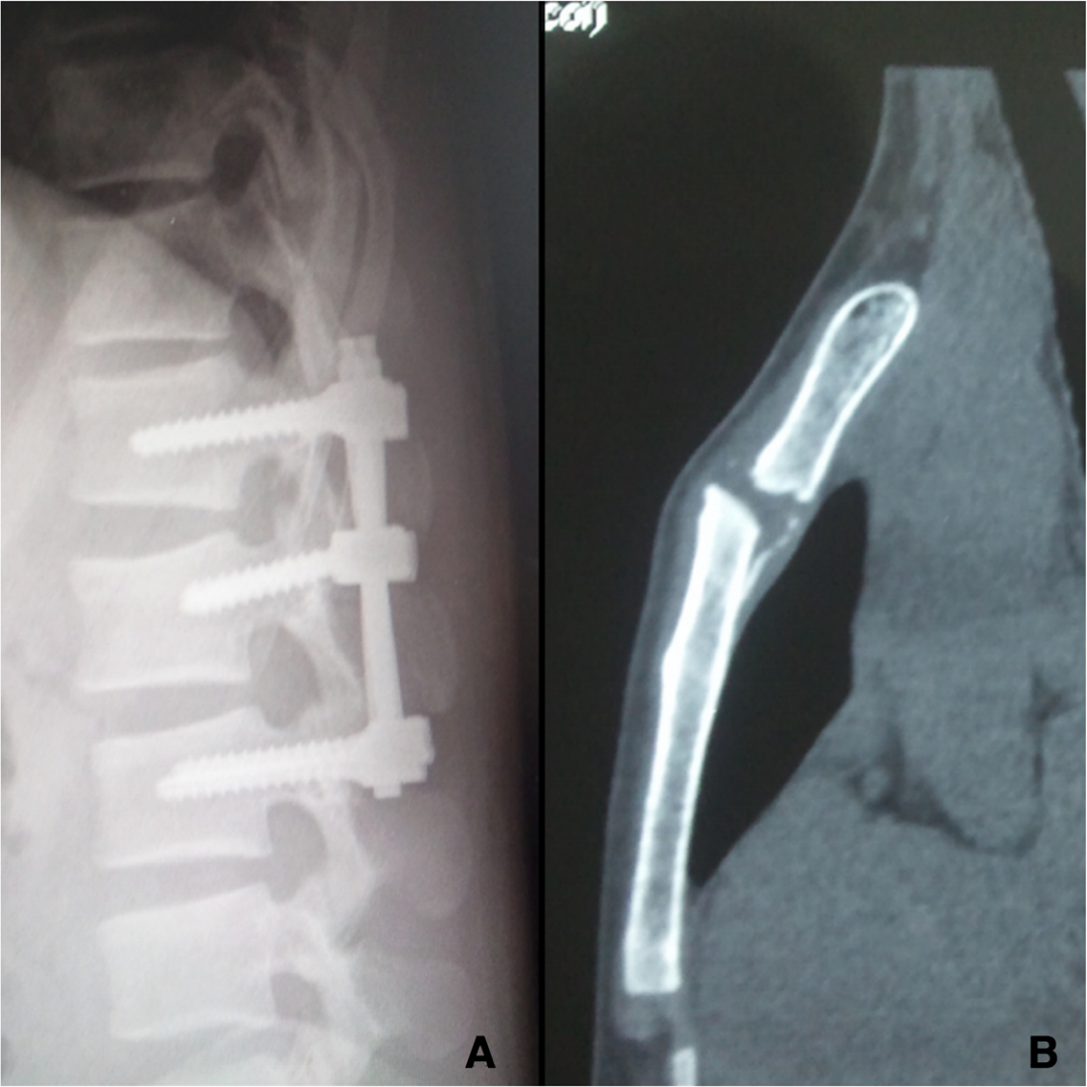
a Lateral radiograph showed fracture healing of the lumber. b sagittal CT scan of the sternum showed anterior dislocation of manubriosternal joint
